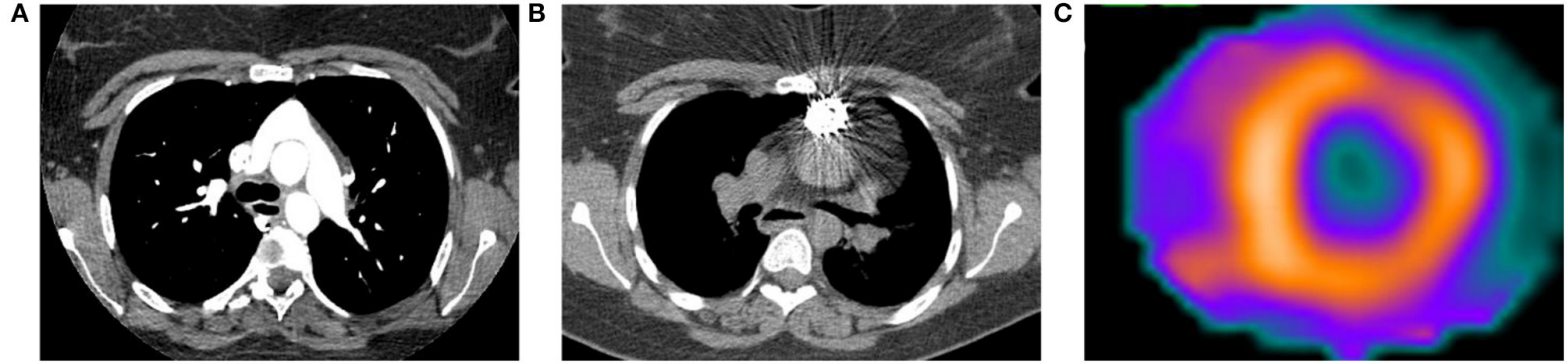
Computed tomography (CT) and ^99m^Tc-sestamibi single-photon emission computed tomography (SPECT) images from a 23-year-old woman with history of D-transposition of the great arteries (D-TGA) status-post repair. (A) Transaxial CT angiogram image demonstrating the characteristic appearance of the pulmonary artery and aorta after repair of D-TGA. (B) Non-contrast CT acquired at time of SPECT shows a stent in the pulmonary artery that was placed after the patient developed severe pulmonary artery stenosis. (C) Short axis SPECT image shows normal radiotracer distribution in the left ventricle with extension of uptake into the visualized portion of the right ventricle, consistent with pulmonary hypertension.
